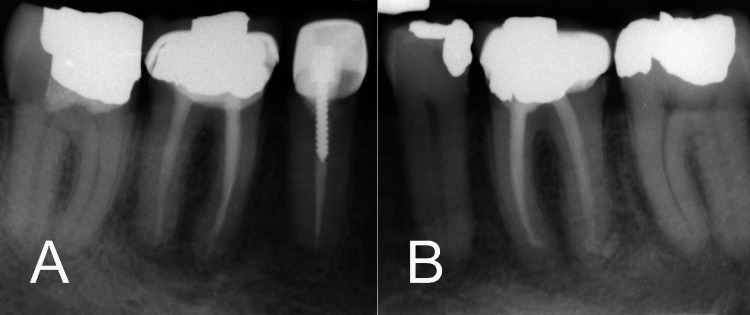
Five-year follow-up radiographs. (A) Tooth #36 showing complete resolution of apical radiolucency, reestablishment of a normal PDL, and absence of symptoms. (B) Tooth #46 revealing similar healing outcomes, with full resolution of the apical lesions and maintenance of the treated tooth structure. PDL: periodontal ligament
